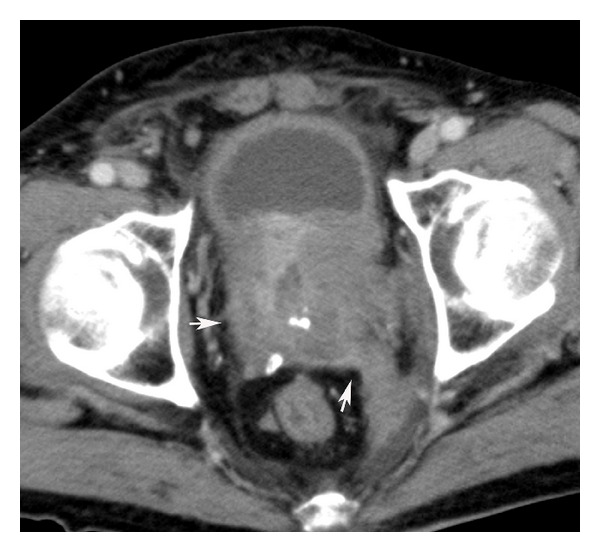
Contrast-enhanced CT demonstrated a large heterogeneous mass (arrow).
